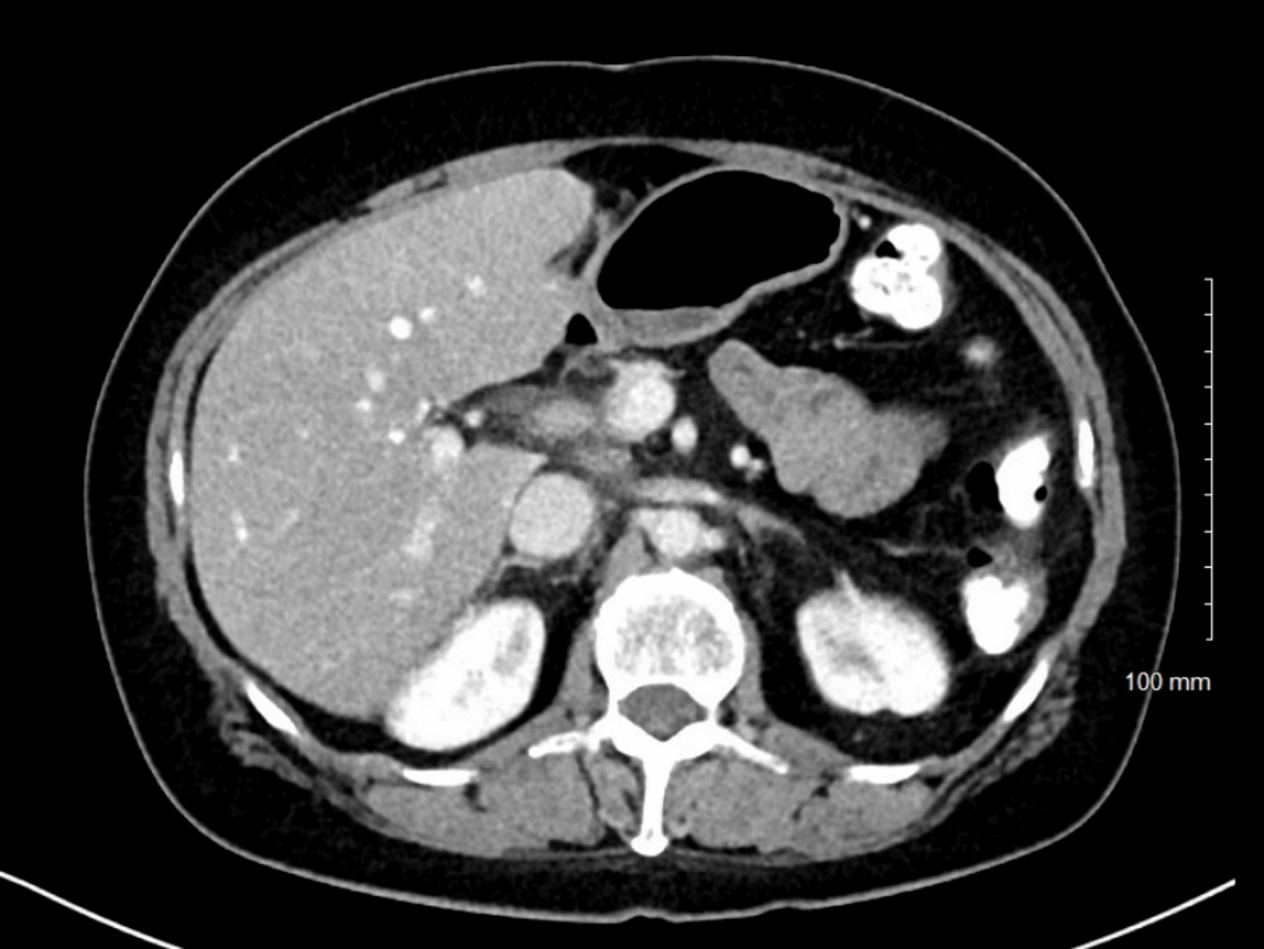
Post-VMAT CT reported as normal VMAT: volumetric modulated arc therapy; CT: computed tomography
